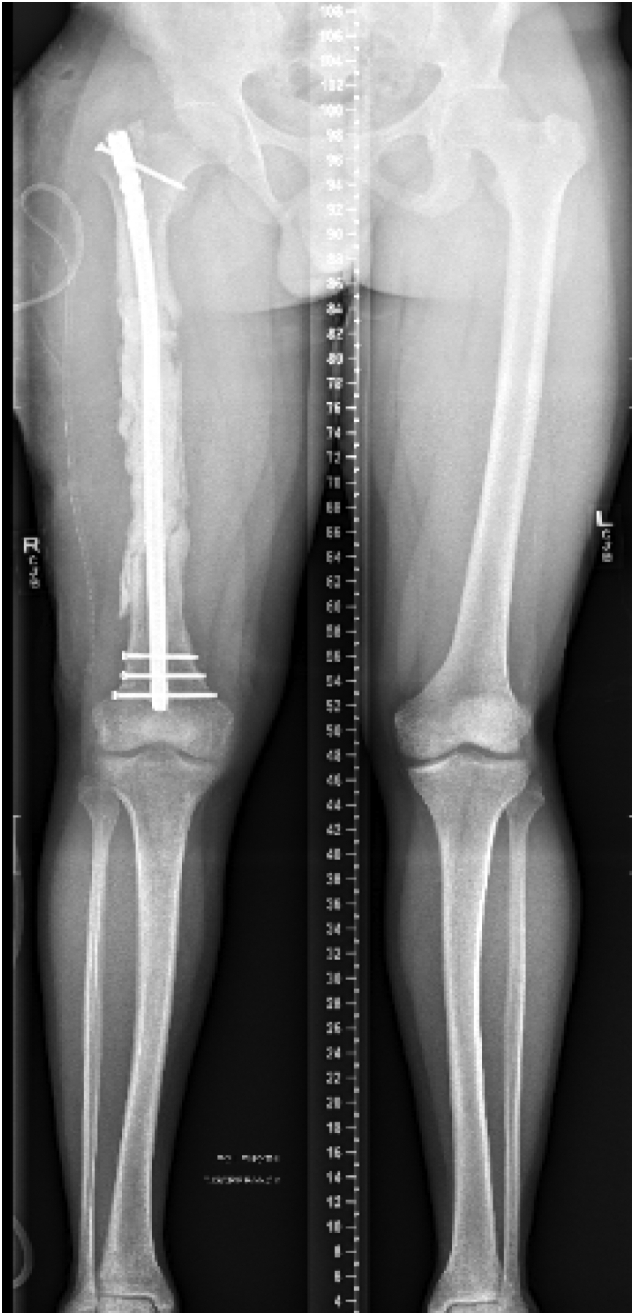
Patient's standing alignment radiograph after the first stage of his Masquelet operation, demonstrating new intramedullary nail and cement spacer in place.
